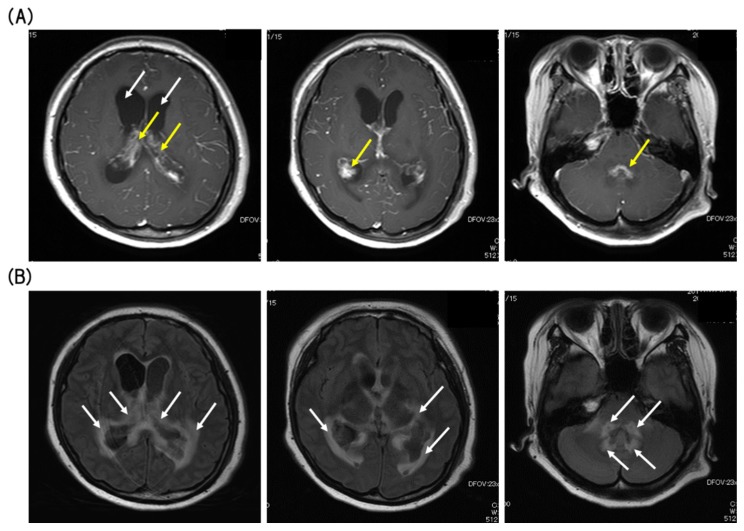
(A) T1-weighted (T1W) axial gadolinium-enhanced magnetic resonance imaging (MRI) showed Bilateral ventricle enlargement (white arrows), and hypertrophy choroid plexus (yellow arrows) with heterogeneous enhancement (axial sections). (B) Fluid-attenuated inversion recovery image (FLAIR) revealed high-intensity periventricular white-matter changes (white arrows) (axial sections).
